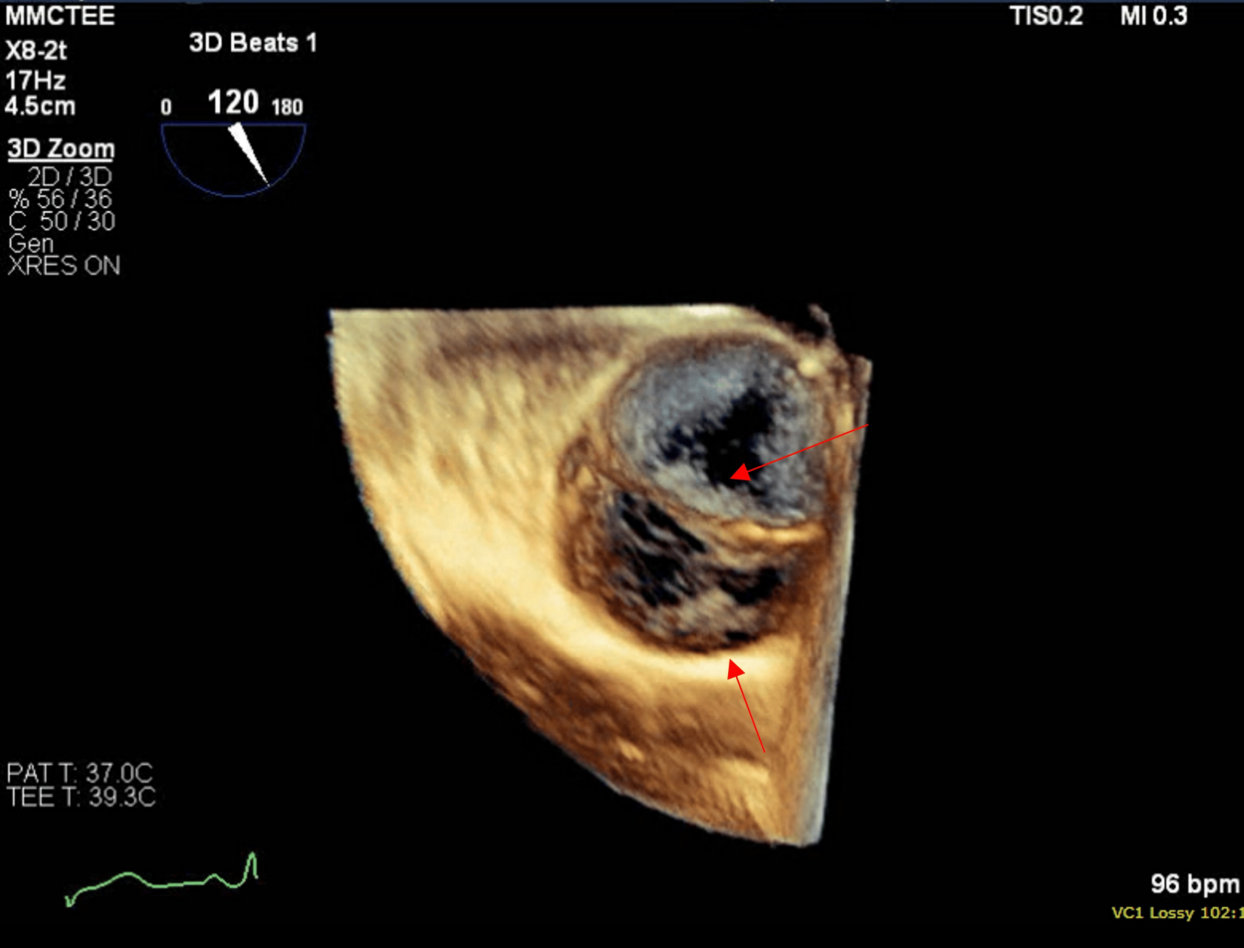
Transesophageal echocardiogram revealing bicuspid aortic valve with valvular vegetation
